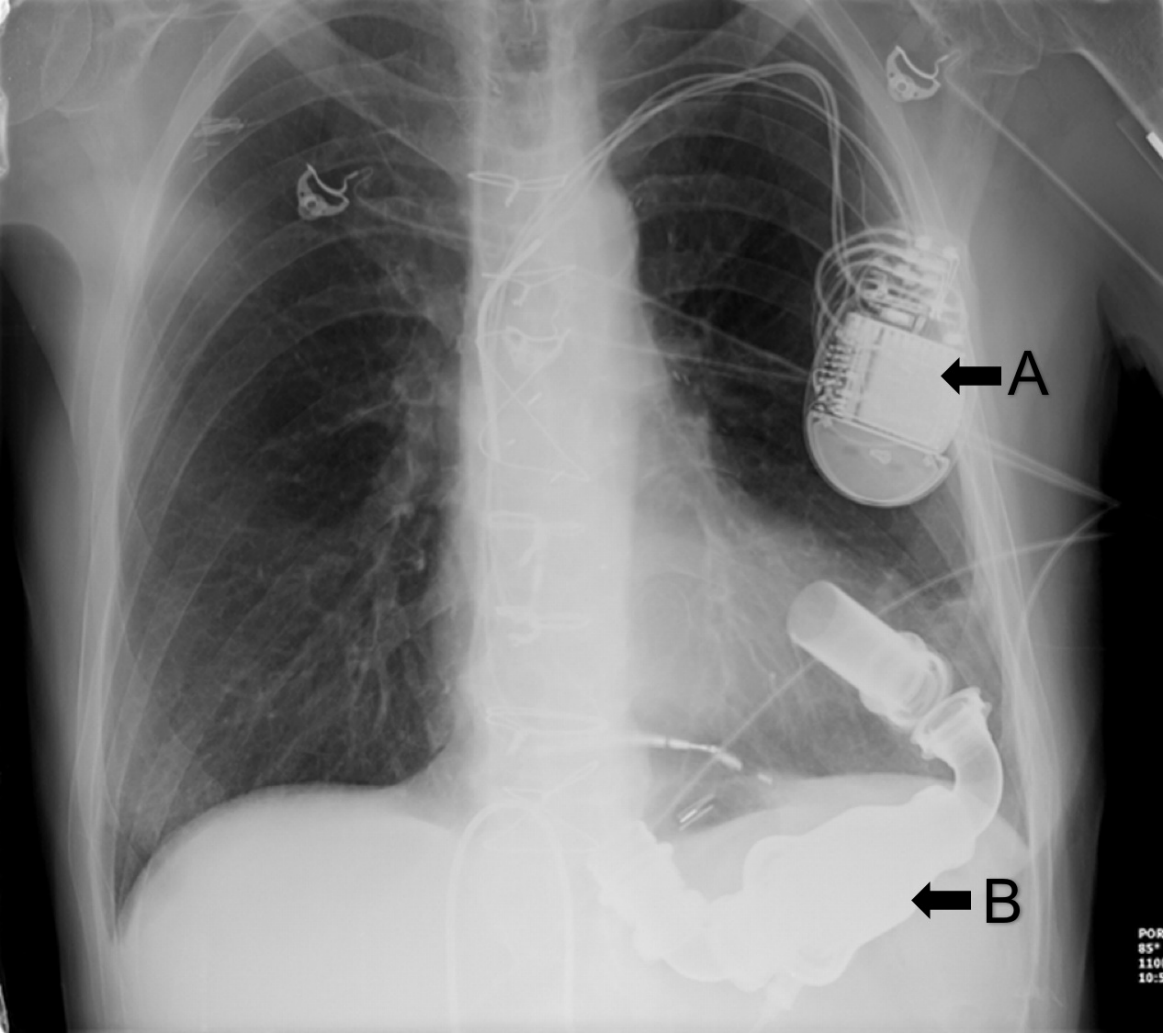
Chest x-ray showing left ventricular assist device (LVAD) A = implantable cardioverter defibrillator (ICD) B = left ventricular assist device (LVAD) Courtesy of: https://emrems.com/tag/lvad/
